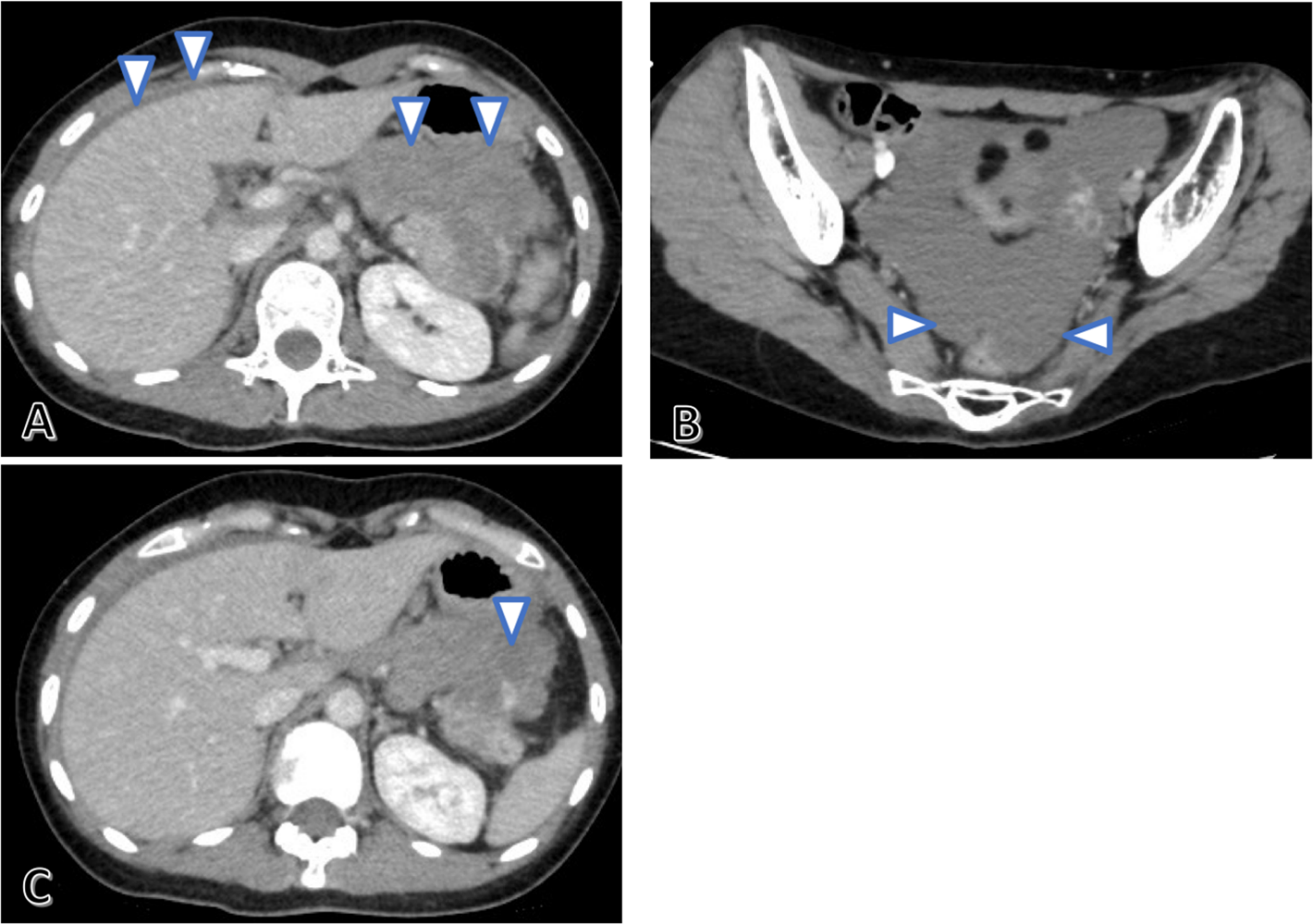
Contrast-enhanced abdominal computed tomography (CT) conducted in the emergency department. Ascites with high CT values (arrow) is observed on the liver surface (a) and in the pelvis (b). Leakage of contrast medium (arrow) is observed from the anterior of the pancreatic tail tumor to the omental bursa (c)
